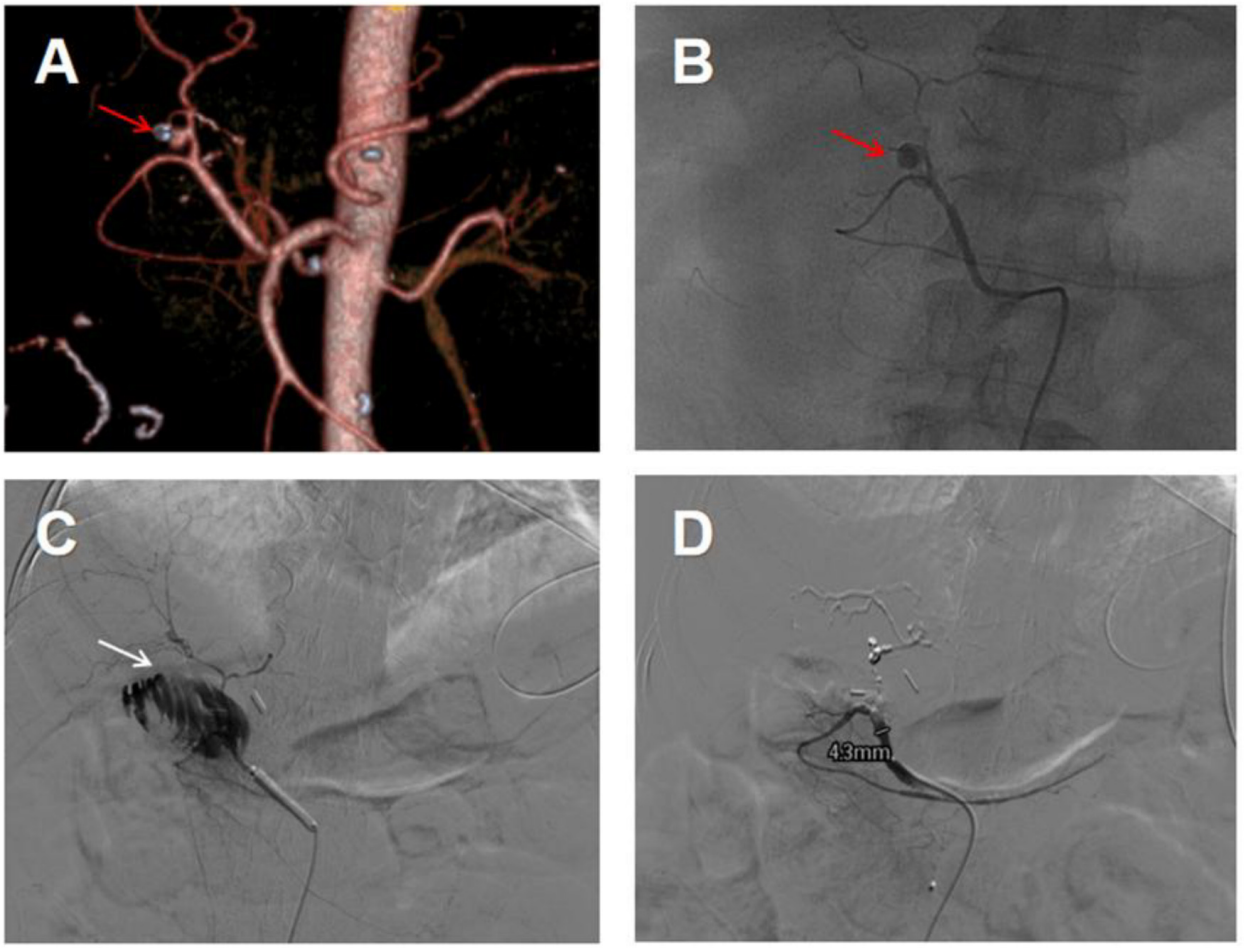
(A) Celiac artery CTA showing a pseudoaneurysm of the proper hepatic artery (red arrow); (B) Celiac arteriography confirming the proper hepatic artery pseudoaneurysm (red arrow); (C) Celiac arteriography confirming communication between the proper hepatic artery pseudoaneurysm and the intestine, with contrast leakage into the intestine (white arrow) and (D) Angiography after coil embolization of the proper hepatic artery showing no residual hepatic artery blood flow.
